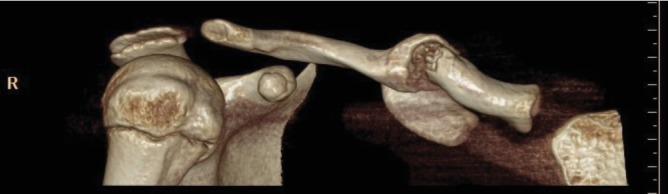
CT scan confirmed the diagnosis of the pseudoarthrosis.
